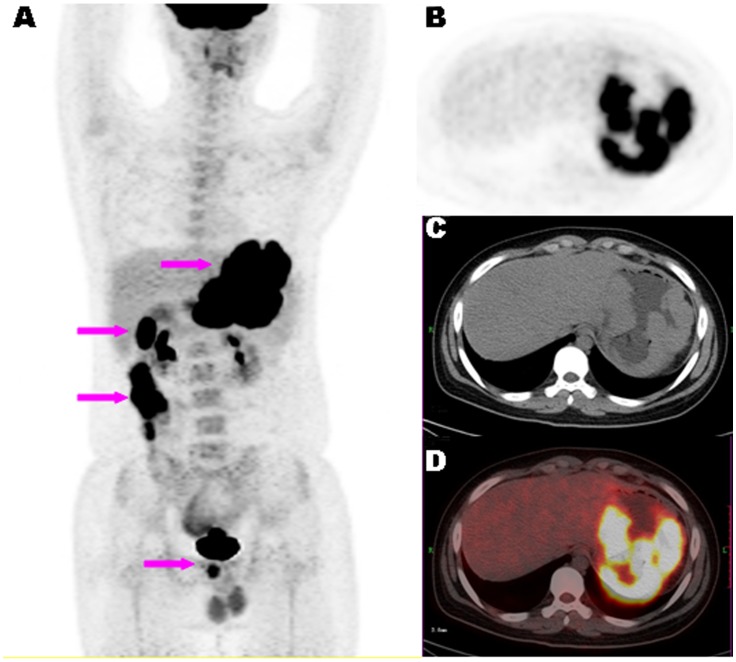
A representative case of ANHL . ^18^F-FDG PET/CT images of a15-year-old male patient with newly diagnosed Burkitt lymphoma. Maximum-intensity-projection view (A) showing multiple hypermetabolic lesions in the gastrointestinal tract (arrows). Axial PET (B), CT (C) and fused PET/CT (D) images showing diffused and irregular thickening of gastric wall with FDG uptake (SUVmax of 18.82).
